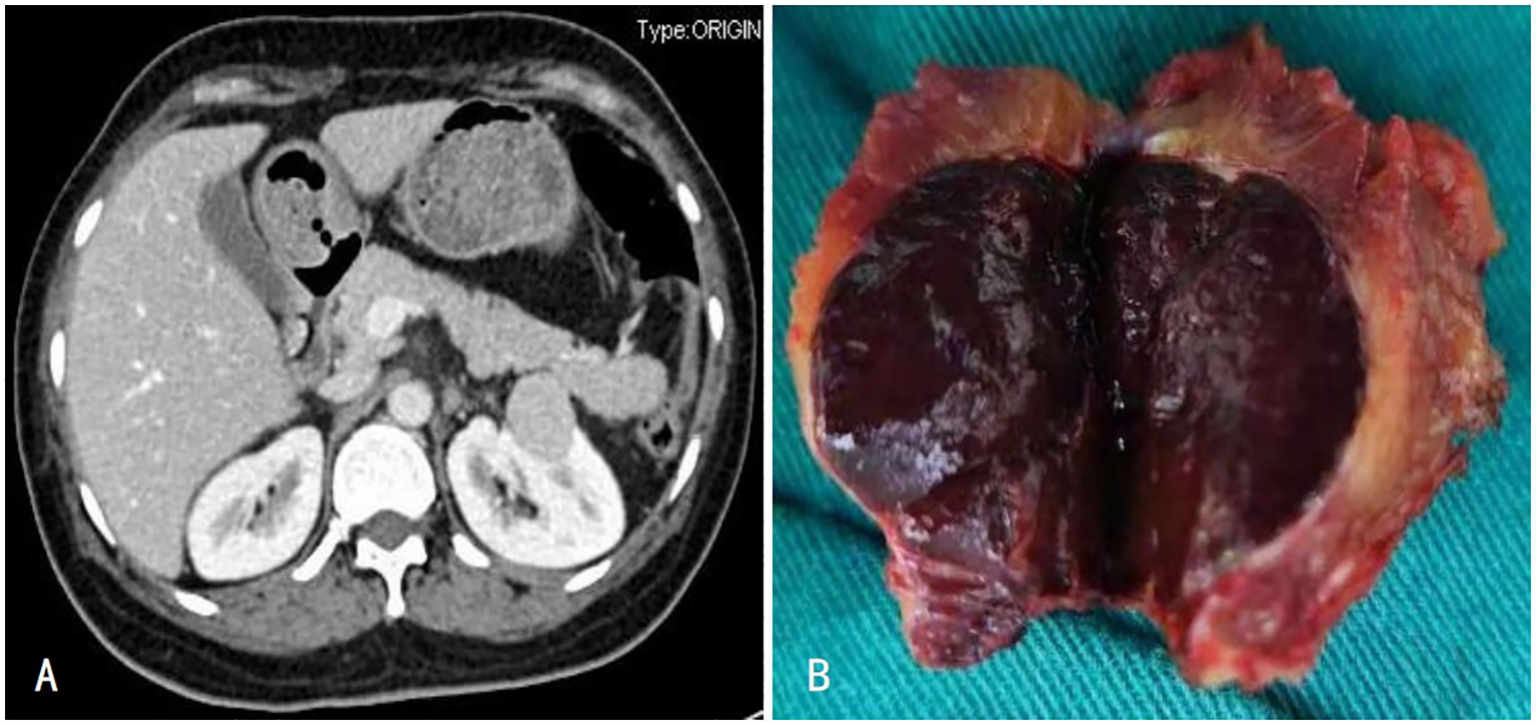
(A) Enhanced CT showed enhancement of the left renal tumor with capsule; (B) The left renal tumor was a dark red mass of tissue.
